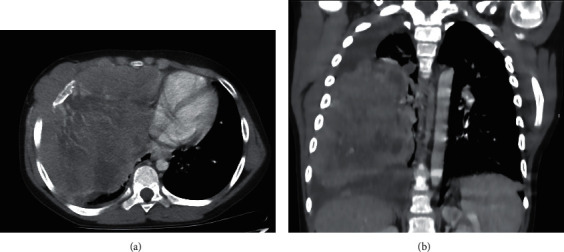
(a, b) A CT chest performed at the initial encounter. The CT chest obtained at the initial encounter revealed a large, heterogeneously enhancing mass occupying the majority of the right hemithorax with peripheral necrosis.
